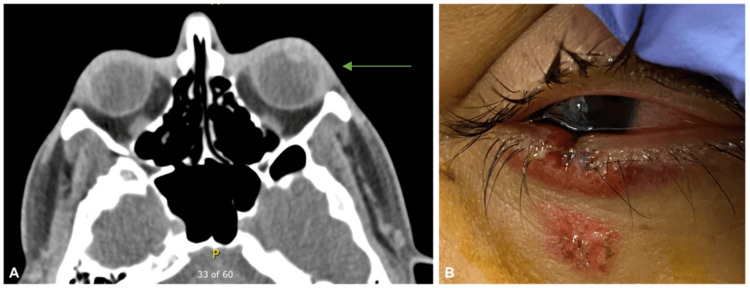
A 35-year-old male (case 4) was struck in the left eye by a projectile from a nearby firework explosion. A: CT scan (sagittal view) showing formed left globe, without evidence of intraocular or intraorbital foreign body (green arrow). B: External photograph of the left eye. There are left lower lid defect and hyphema CT: computed tomography
